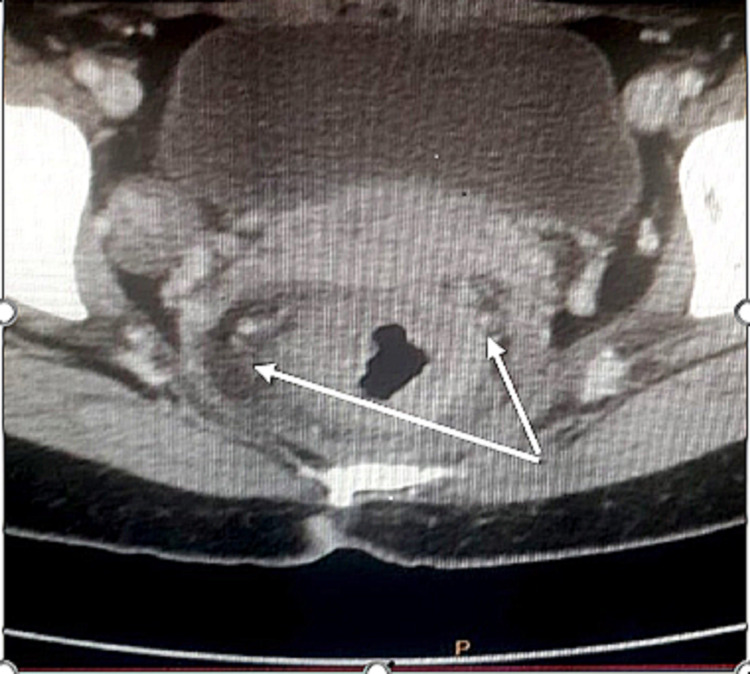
Computed tomography (CT) indicates tumor-like thickenings of the rectum
